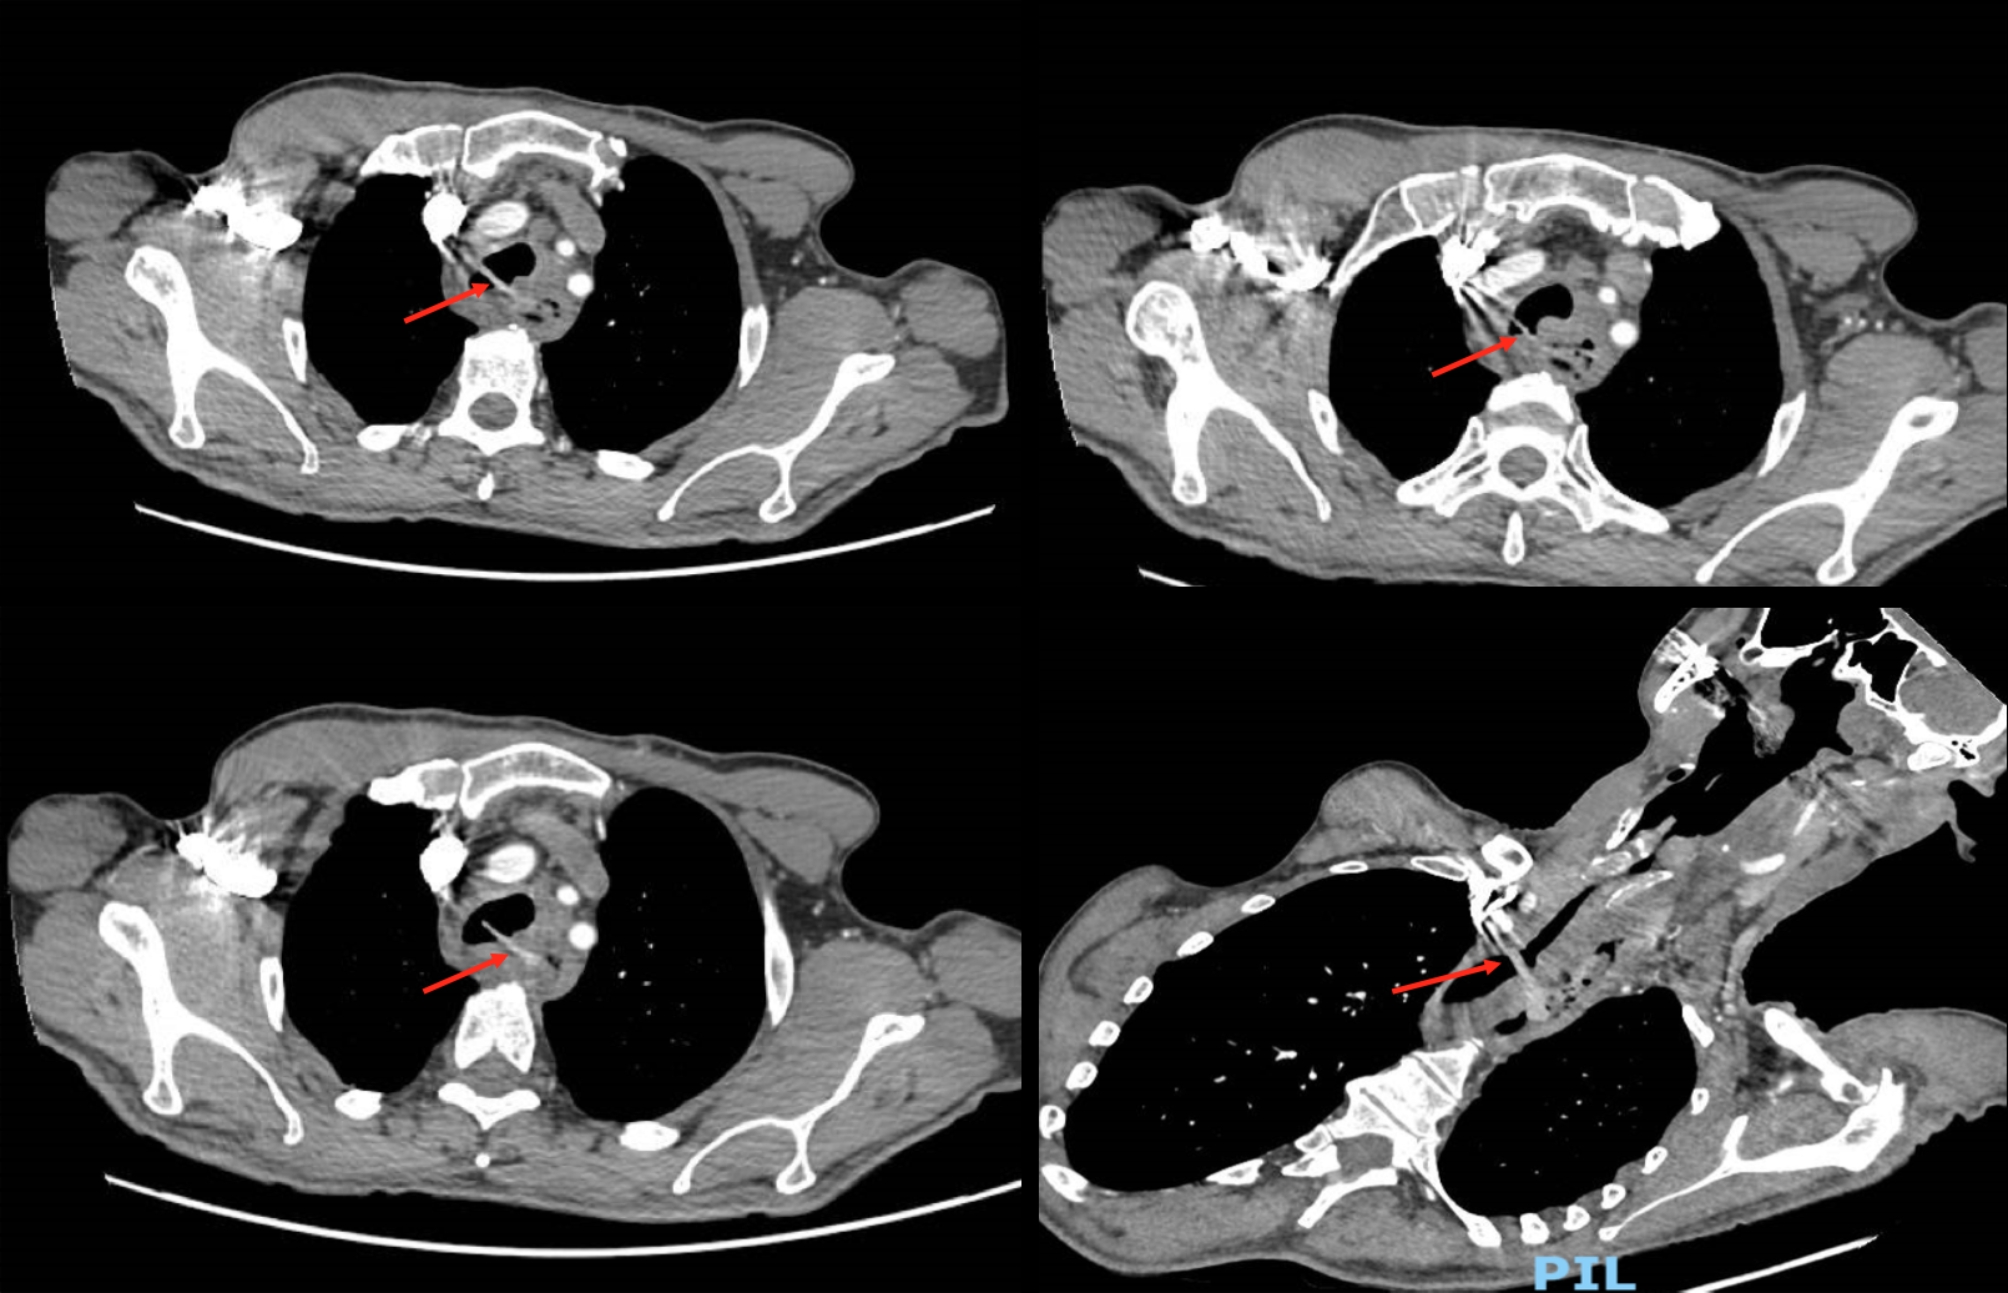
Axial slices of CT scan with contrast. Shows a linear hyperdense shadow extending from oesophagus to proximal trachea within a tracheo-oesophageal fistulous tract at the level of T2 vertebrae
